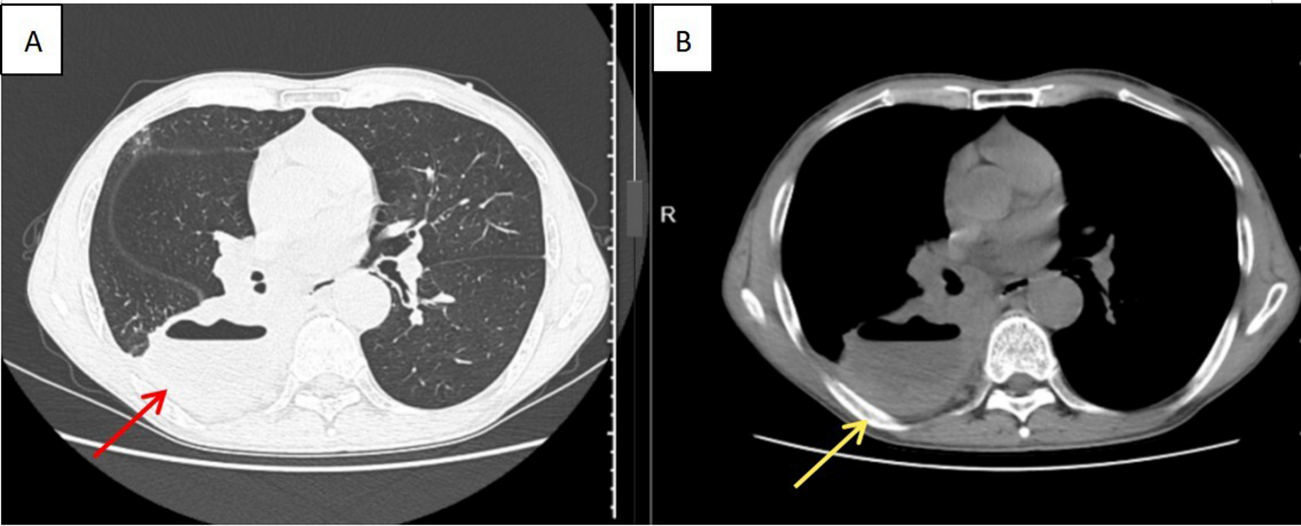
Enhanced chest CT demonstrated a right lung mass with mediastinal and right hilar lymphadenopathy. (A) (lung window) clearly delineates the morphology and margins of the lesion within the pulmonary parenchyma; (B) (mediastinal window) shows the enlarged mediastinal lymph nodes.
